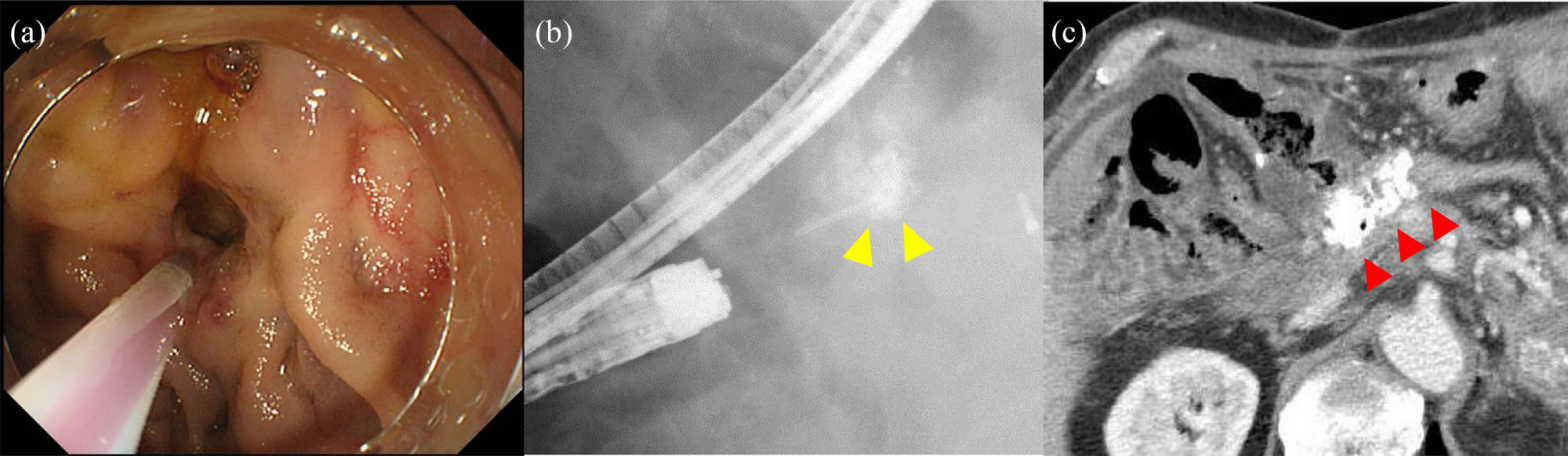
Endoscopic glue injection therapy of case 1: (a) We punctured varix with a 20‐gauge needle and recognized backflow. (b) We injected the cyanoacrylate (CA) with iodized poppy oil into varix. (c) Computed tomography after the procedure showed that the CA was retained in the ectopic varices around the choledochojejunostomy
